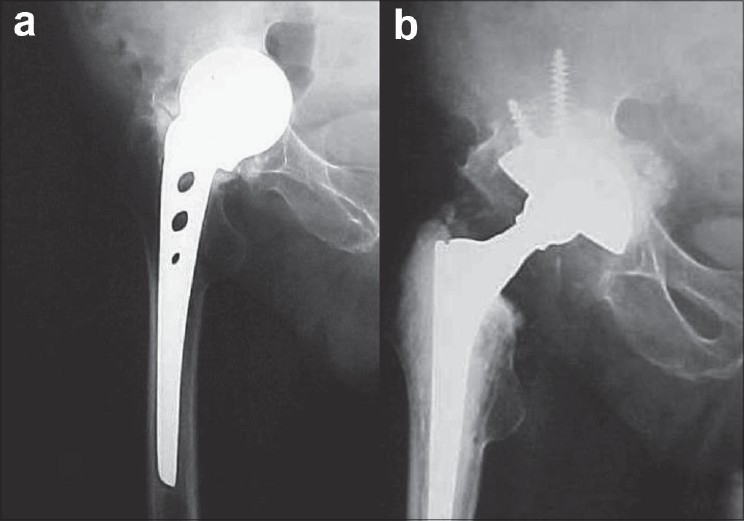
(a) Preoperative radiograph of a failed hemiarthroplasty with acetabular erosion and protrusio, (b) Two-year follow-up X-ray showing incorporation of morsellized allograft
